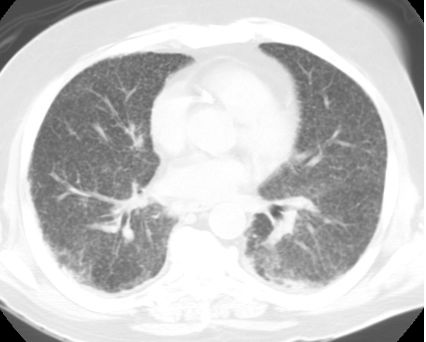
The patient's CT demonstrating bilateral, miliary interstitial infiltrate pattern.
